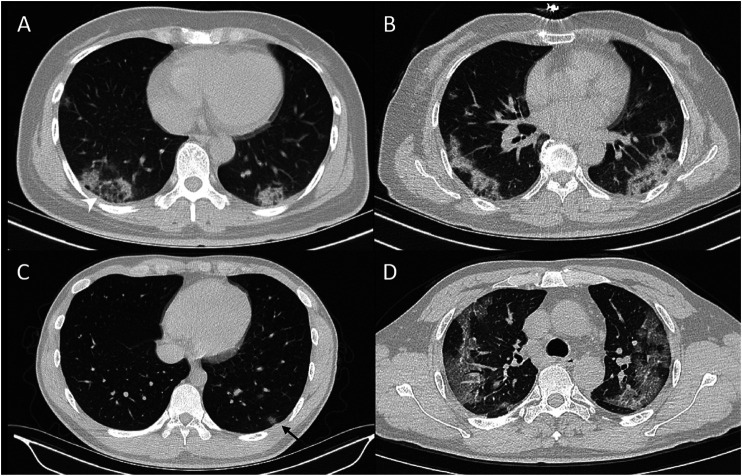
CT signs of reversed hollow sign (A), bilateral distribution of lesion (B), and nodule with hollow sign (C), and crazy-paving sign (D).
